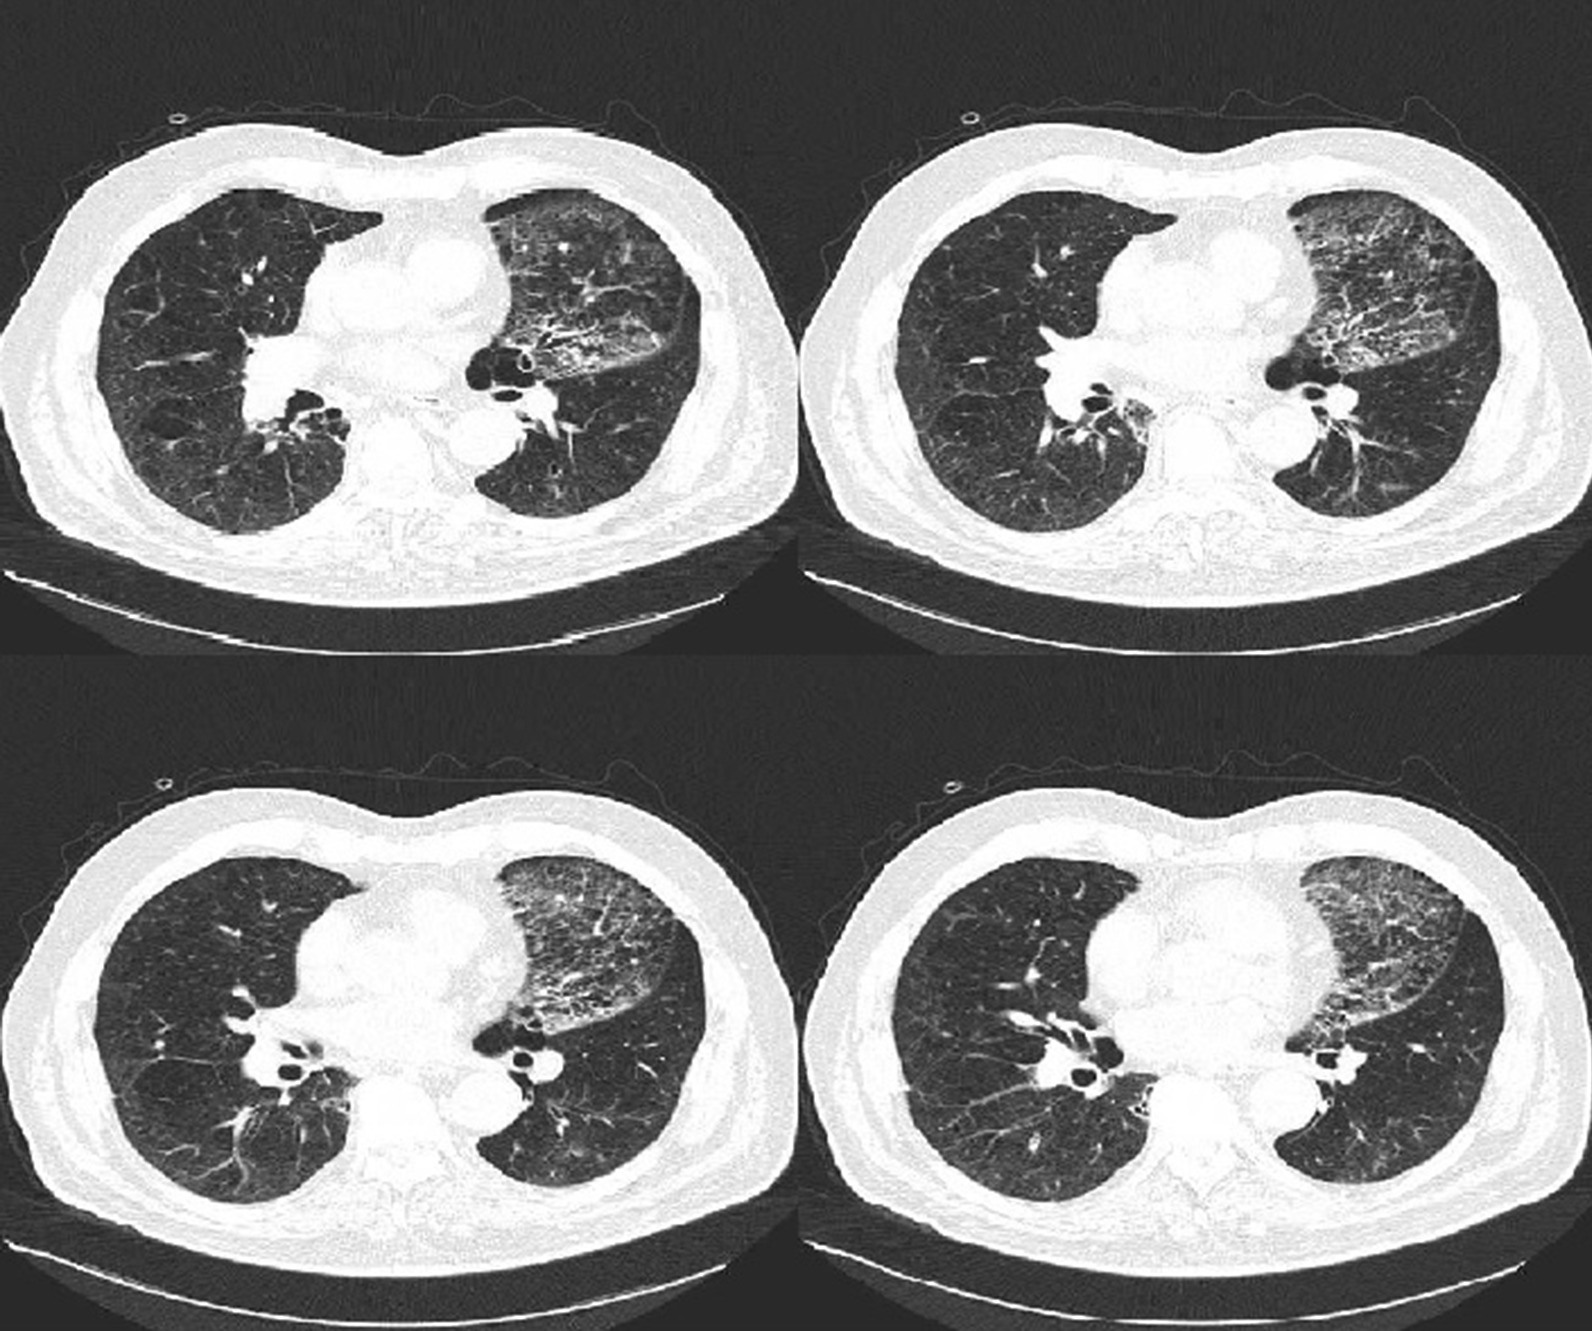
Chest tomography performed on August 2019, after the treatment. The CT scan shows a parenchymal pulmonary inflammatory response of the anterior segment of the left UL, with consequent reduction of lung volume
